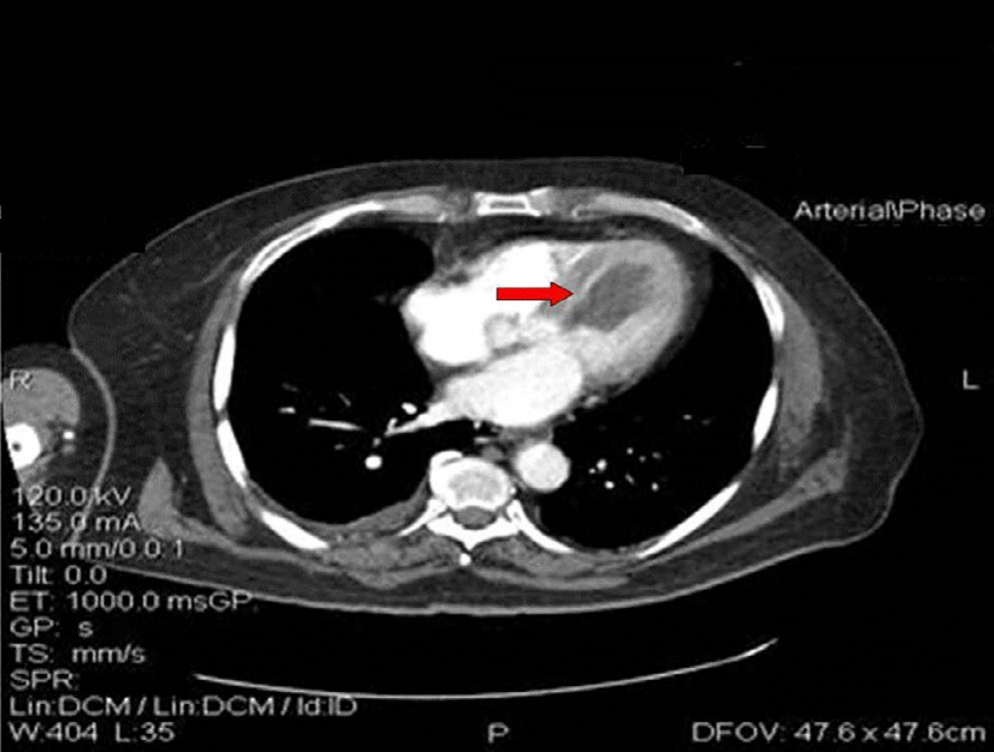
CT‐scan of the thorax: Presence of a tumor in the left chamber (red arrow)
